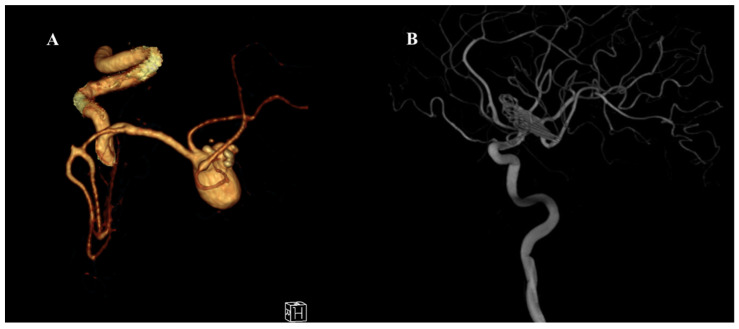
Preoperative and postoperative angiographic images of a giant middle cerebral artery (MCA) aneurysm. (A) Three-dimensional CT angiography reconstruction demonstrating a giant MCA bifurcation aneurysm with multilobulated morphology and complex neck anatomy. (B) Postoperative digital subtraction angiography (DSA) image showing complete occlusion of the aneurysm following surgical clipping using the picket-fence technique. The parent vessels and MCA branches remain patent without evidence of residual aneurysm or stenosis.
